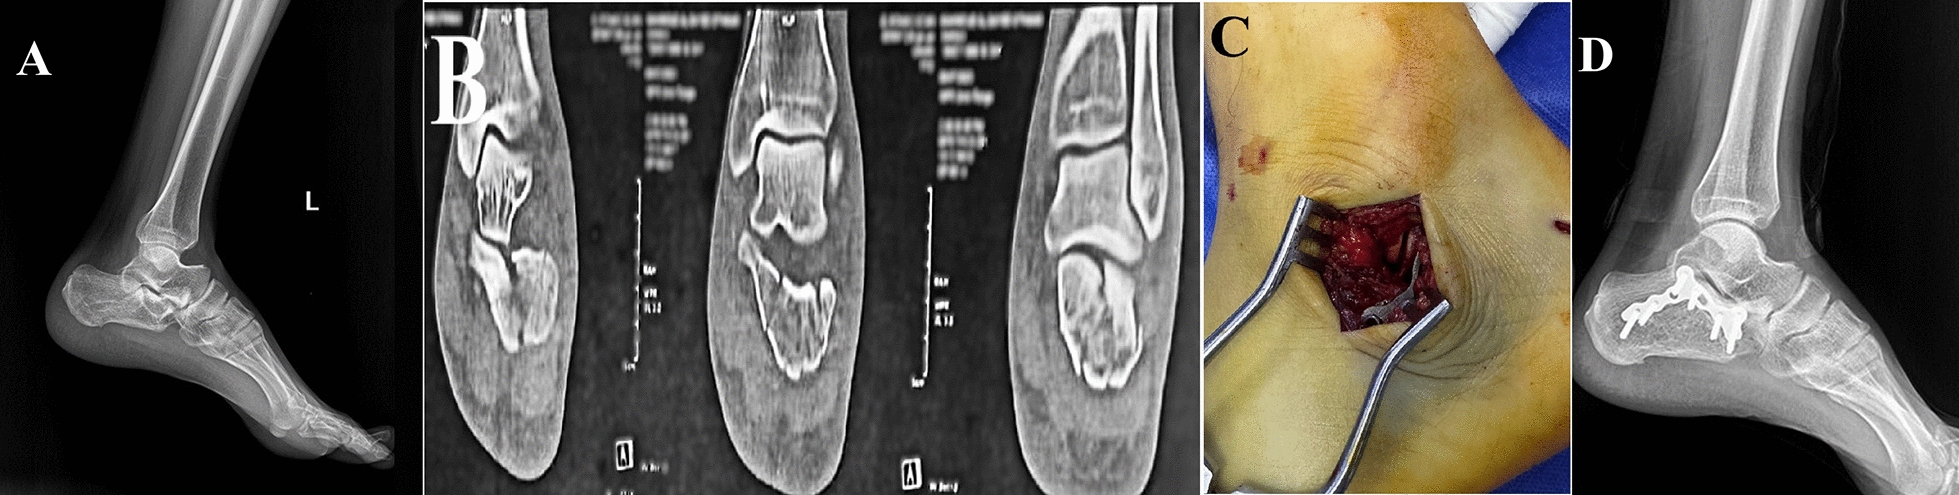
Male patient 35 years old having Sanders type III fracture treated by open reduction and internal fixation through sinus tarsi approach (STA). A Preoperative radiograph showing intraarticular calcaneal fracture with apparent joint depression. B Preoperative computed tomography showing Sander’s type III fracture. C Intraoperative demonstration of the STA. D Plain radiographs at one-year follow-up showed fracture union and maintained articular surface
